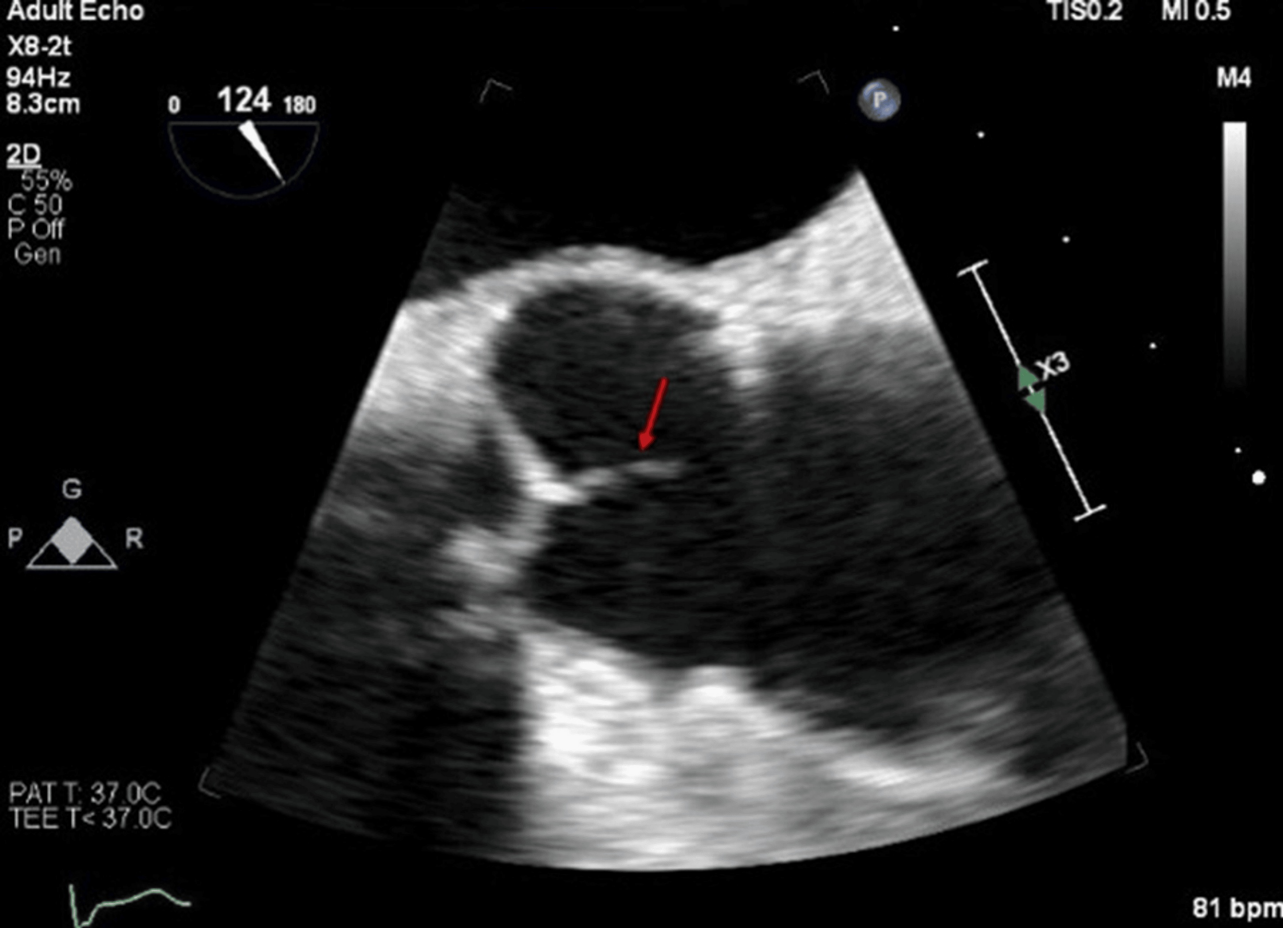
Transesophageal echocardiograph showing a small filamentous echo density visualized on the aortic side of the aortic valve, which was consistent with Lambl’s excrescence (red arrow)
